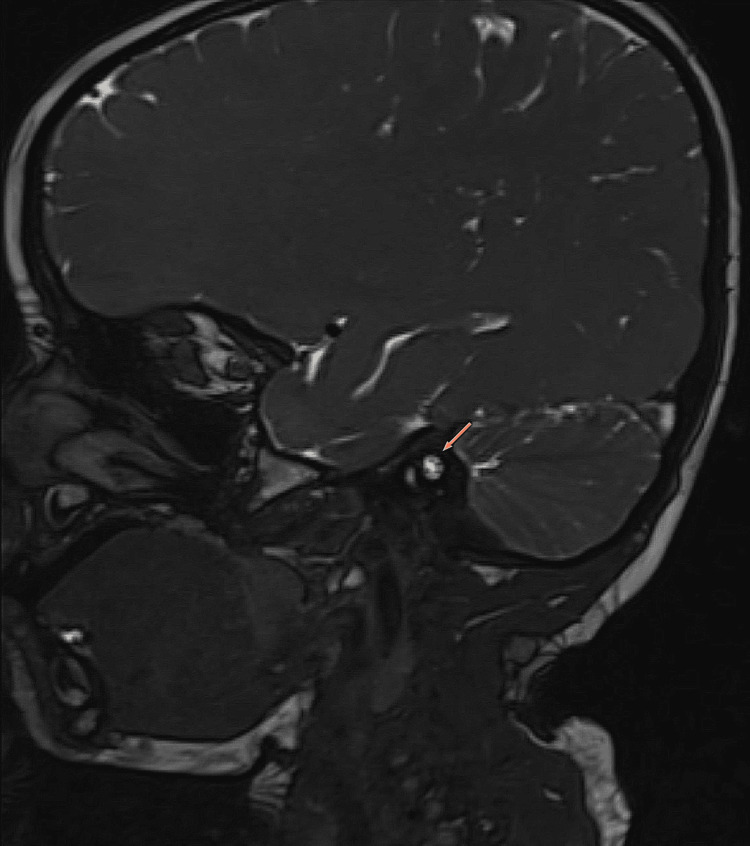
Magnetic resonance image of the left temporal bone Normal appearance of the cochlear nerve seen within the internal auditory canal (arrow) in the antero-inferior quadrant with facial nerve seen in the antero-superior quadrant. Superior and inferior vestibular nerves are also visualized in the postero-superior and postero-inferior quadrants respectively.
